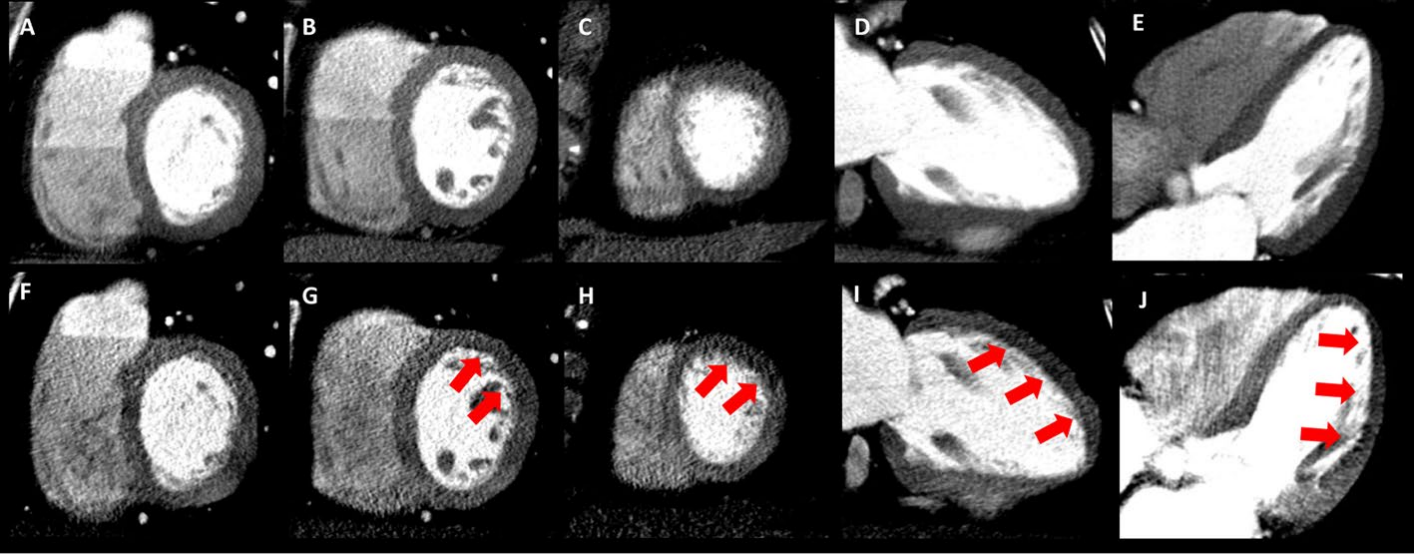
CT-MPI Rest-Stress visual analysis. CT-MPI in Rest (A–E) and Stress (F–J) in short axis, 2-chamber and 4-chamber multi-planar planes images with 5–7 mm slice thickness, display 200–300 HU window width and 100–150 HU window length. Perfusion defects on post dipyridamole stress CT-MPI in mid and apical anterior/anterolateral segments in a single patient (red arrows on G–J). The extent and severity of stress perfusion defect was scored as 1 for mid anterior segment, 2 mid anterolateral segment, 3 for apical anterior segment and 1 apical lateral segment. This corresponded to summed stress score of 7, summed rest score of 0 and sTPD 10%, iTPD 10%. *TPD* total perfusion deficit
